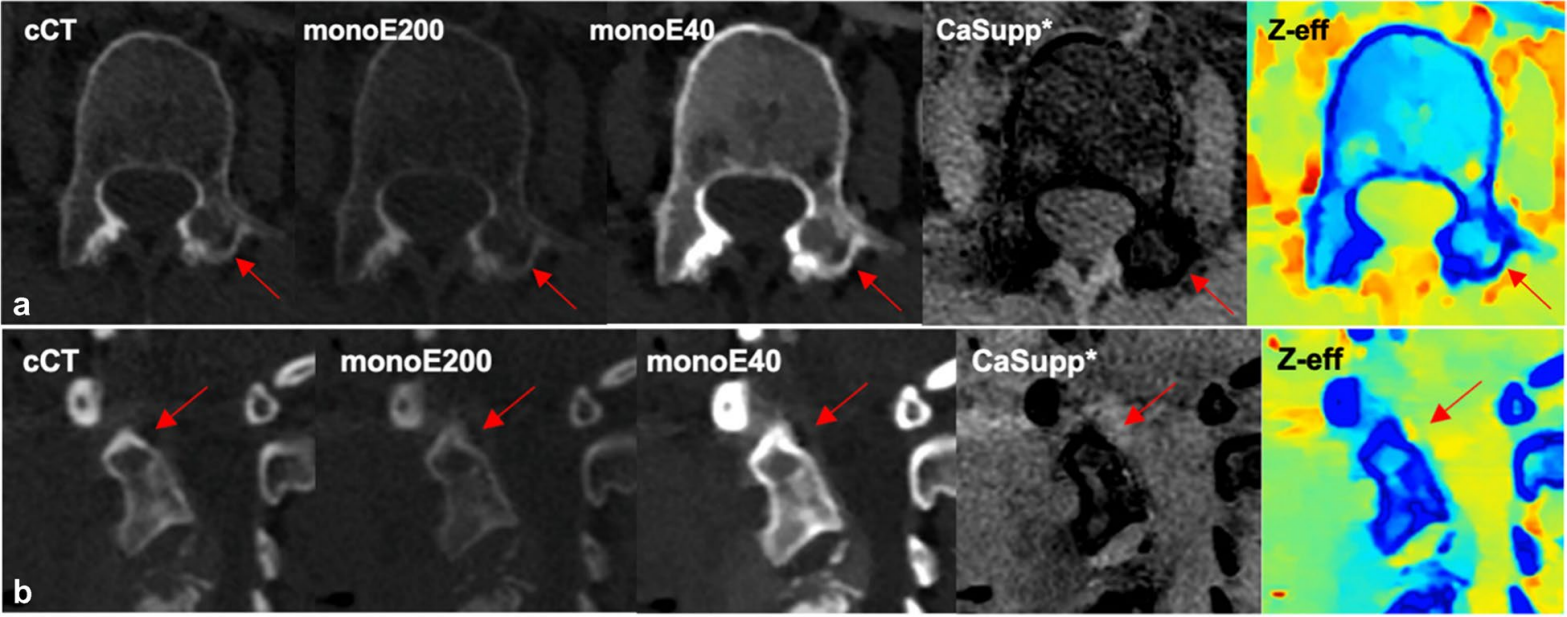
Conventional CT and dual-layer CT reconstructions of two metastases from different patients in the left pedicle of L2 and the odontoid process of C2 that were only detected by observer B on monoE40 and monoE200 (a), and monoE40 (b), respectively. *Abbreviations: cCT, conventional CT; monoE200, monoenergetic 200 keV; monoE40, monoenergetic 40 keV; CaSupp, Calcium suppression; Z-eff, Z-effective. *Calcium suppression index of 55*
